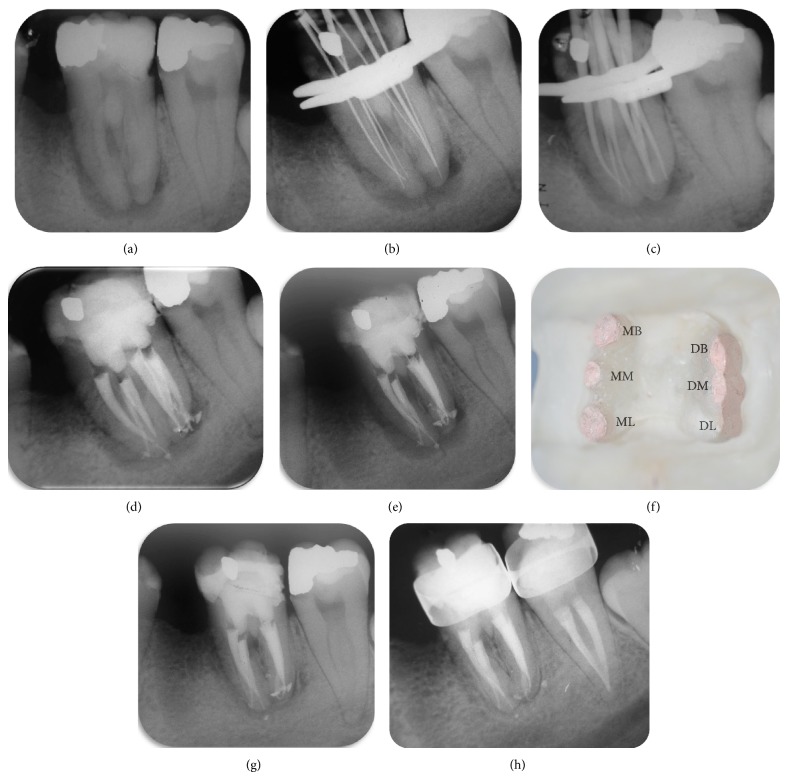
Preoperative radiograph shows normal root canal anatomy and apical lesion (a); radiograph to determine working length (b); radiograph shows gutta-percha cones placed in canals (c); postobturation radiograph shows 6 root canals and independent apical foramina ((d) and (e)); clinical view of pulp chamber after root canal treatment (f); periapical repair 6 (g) and 18 months after treatment (h).
